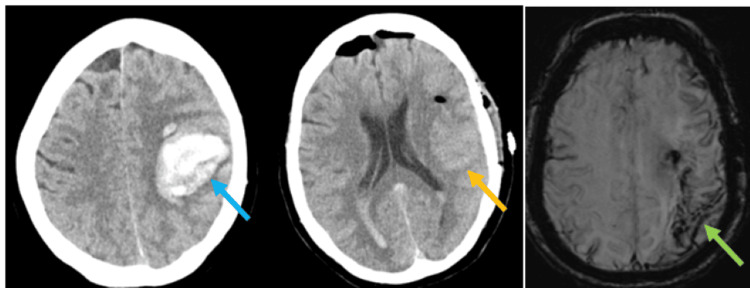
Left temporal lobar haemorrhage (blue arrow) that then underwent haematoma evacuation (orange arrow); the susceptibility weighted MRI shows multiple microbleeds and cortical siderosis in the left parieto-occipital region (green arrow)
